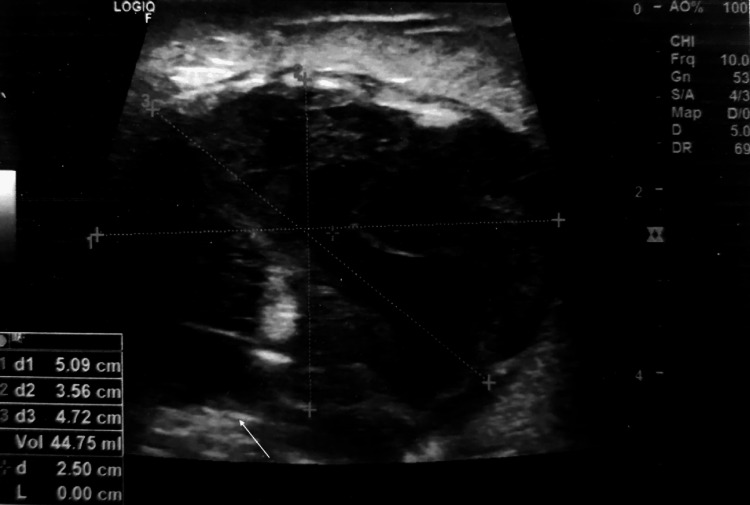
Sonographic marking of a tumor with a surgical clip seen in situ (white arrow).
